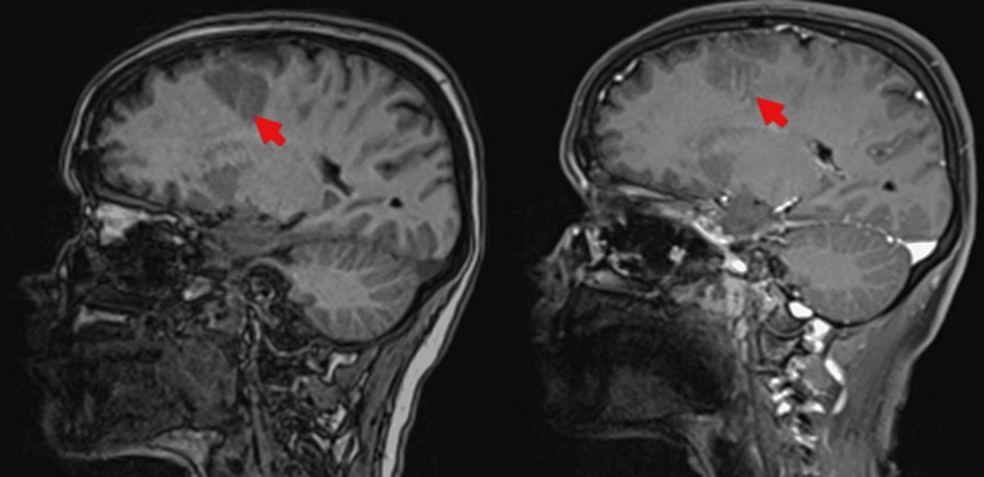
Sagittal T1-weighted image with contrast showing contrast enhancement of the lesions.
